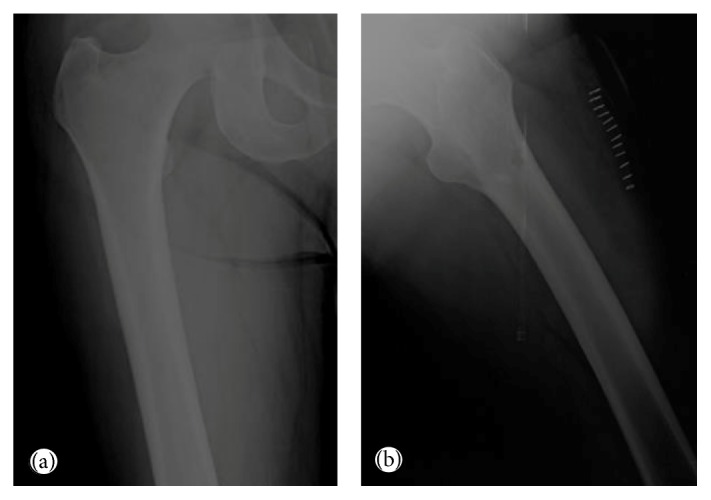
Anteroposterior radiographs of both femurs. (a) Mixed lytic-sclerotic lesions on the right femur. (b) Mixed lytic-sclerotic lesions on the left femur and a lytic destructive process with eccentric localization and a narrow zone of transition at diaphysis of the left femur extending to the left subtrochanteric region.
